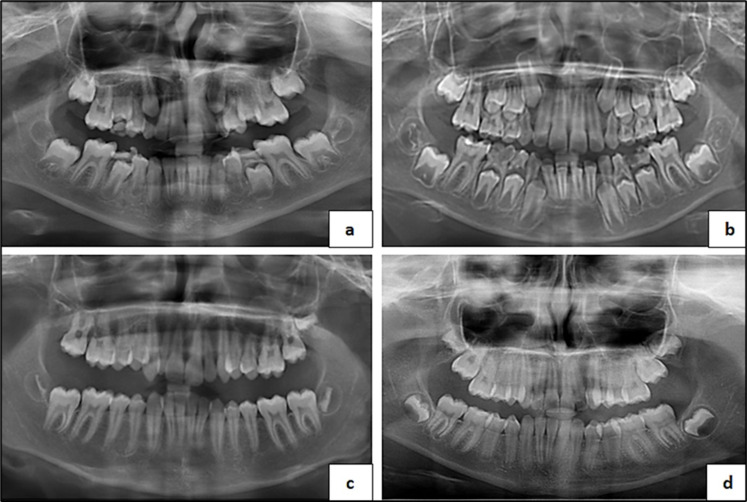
Panoramic radiographs showing third-molar germs at different ages: (a) 9 years, (b) 10 years, (c) 11 years and (d) 12 years.
